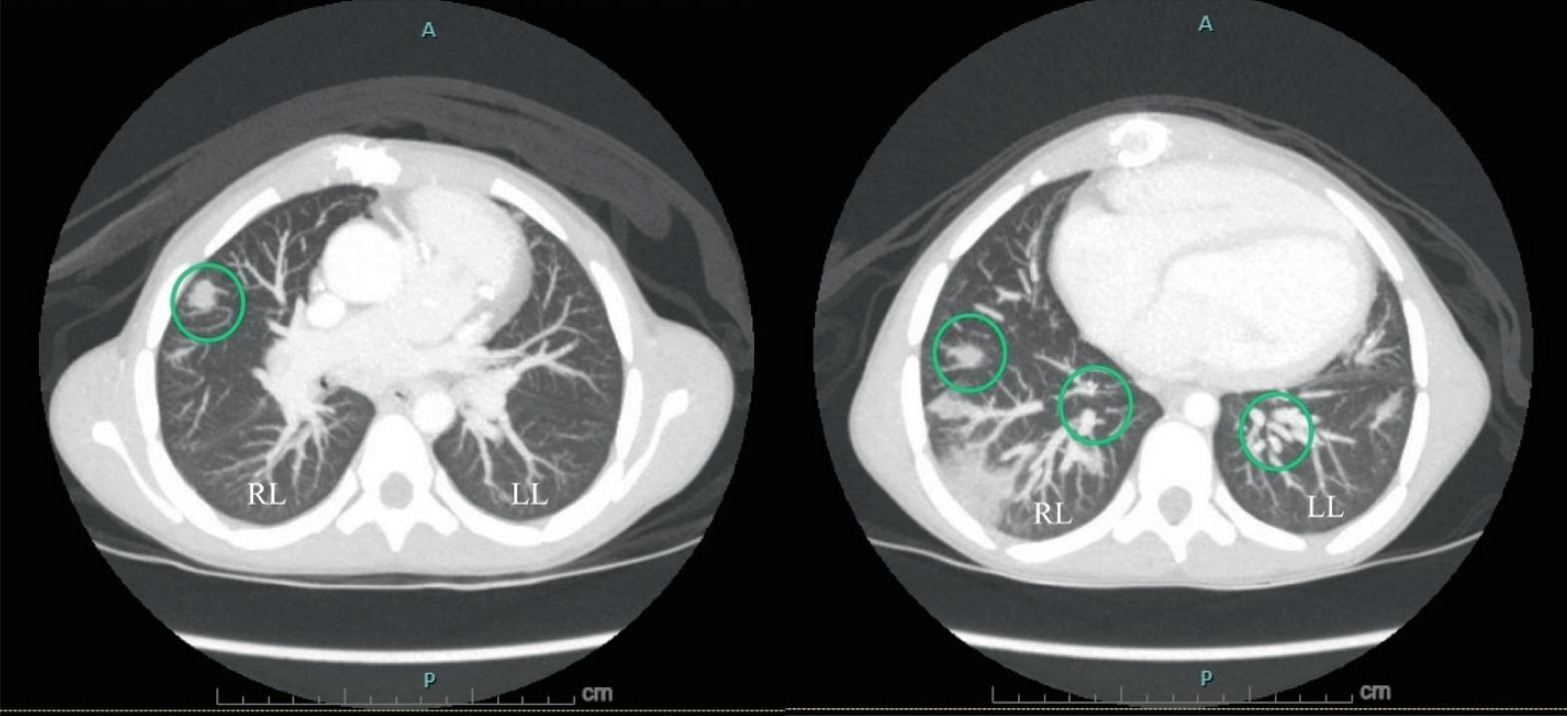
Contrast CT scan of the chest in axial plane showing bilateral multifocal septic pulmonary emboli (circles). (RL = right lung, LL = left lung).
